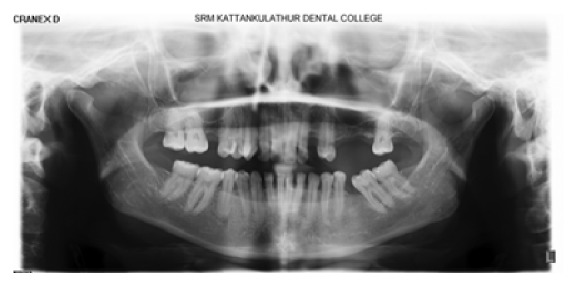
Orthopantomograph shows coronal radiolucency in #14 and #15 with multiple edentulous spaces. No periapical radiolucencies were evident.
